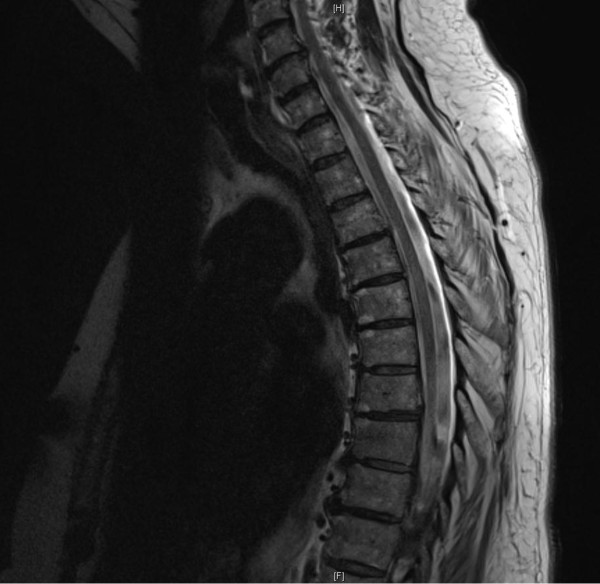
Sagittal magnetic resonance imaging of the thoracic spine on day 1 after the abdominal surgery and epidural anesthesia. Note the absence of canal compromise, edema, and bleeding.
